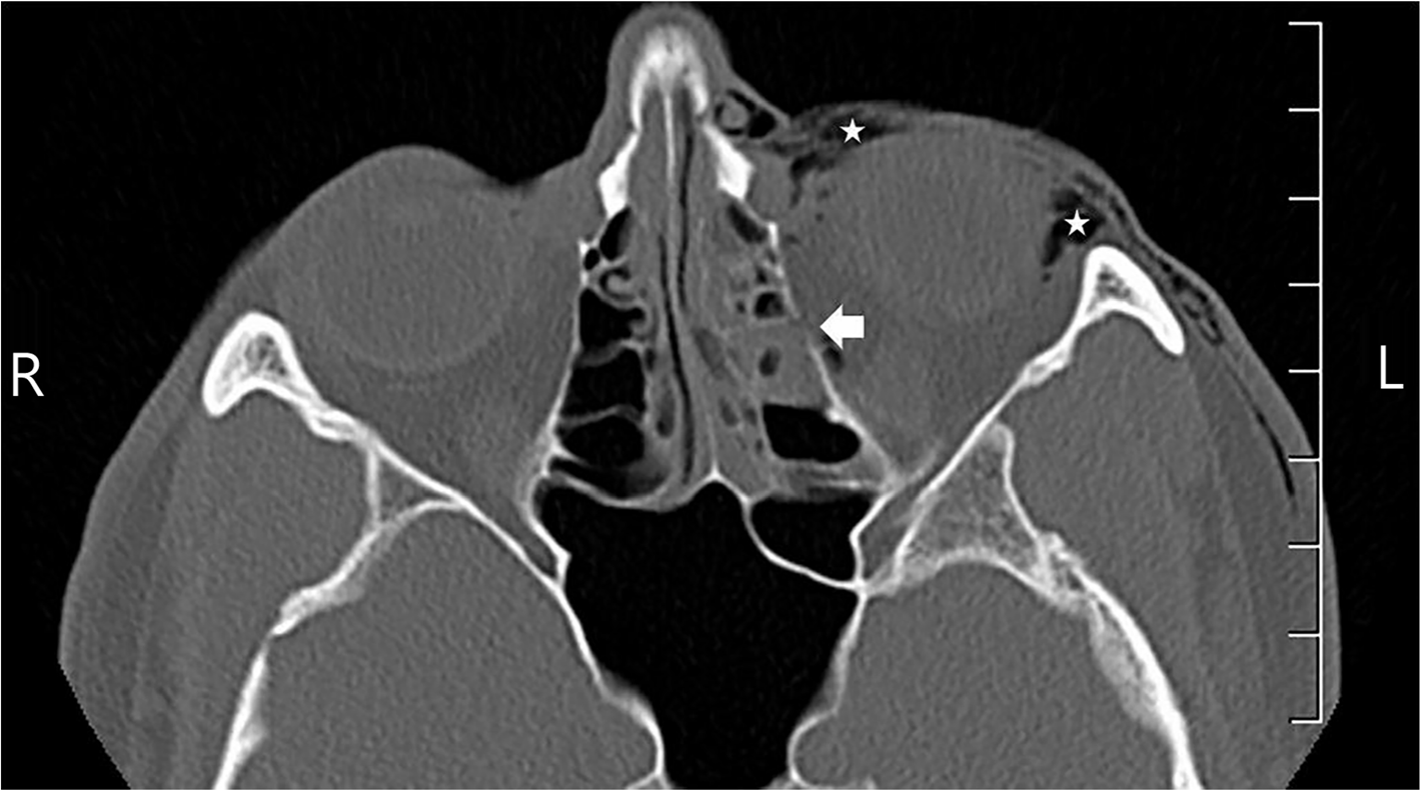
Axial noncontrast CT of the orbits. Axial CT of the orbits demonstrates a 5-mm nondisplaced fracture through the medial wall of the left orbit, which results in communication with the adjacent ethmoid air cells (thick arrow). Subcutaneous emphysema is present within the pre and postseptal regions and anterior soft tissues (starred). A single locule of air was noted within the intraconal left orbit
